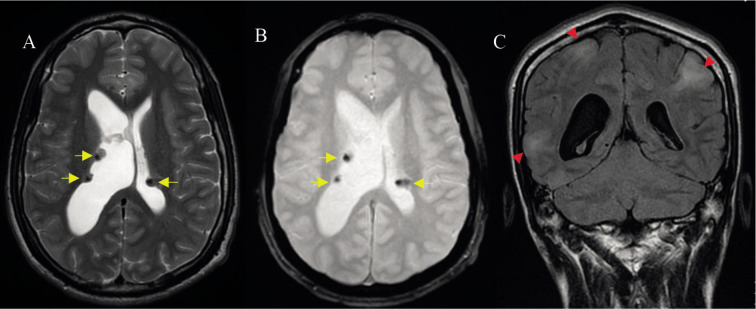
Brain MRI study. Image A and B: Axial T2 cut (A) and gradient (B) where the subependymal nodules (yellow arrows) in both lateral ventricles are identified with a drop in signal intensity in the gradient sequence due to calcification. Image C: Coronal T2 FLAIR cut, where several hyperintense cortical-subcortical lesions compatible with cortical tubers are identified (red head arrows).
